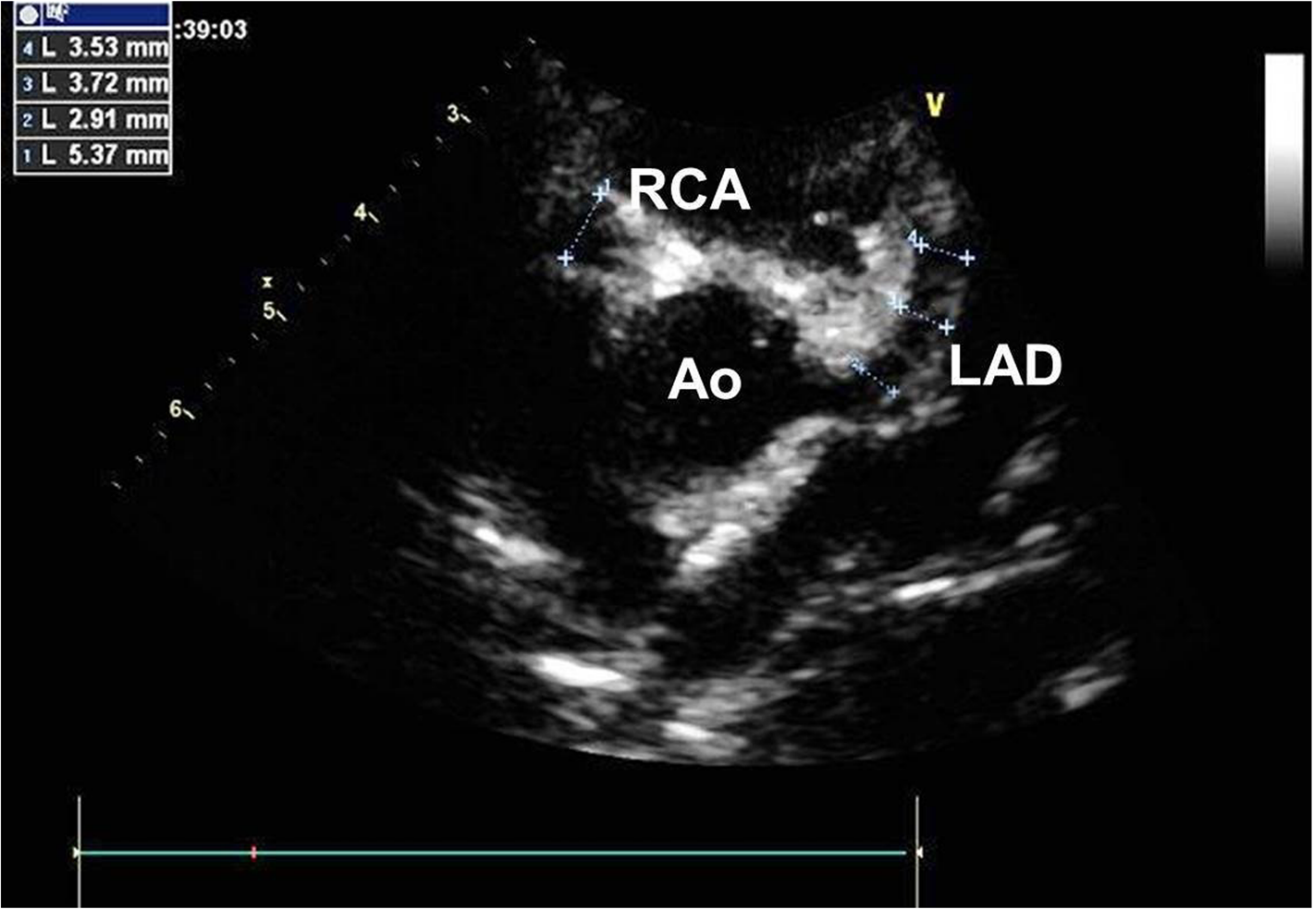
Transthoracic echocardiography of the patient showing the fusiform aneurysm of the right coronary artery and the ectatic left anterior descending coronary artery. Ao: aorta; RCA: right coronary artery; LAD: left anterior descending coronary artery
